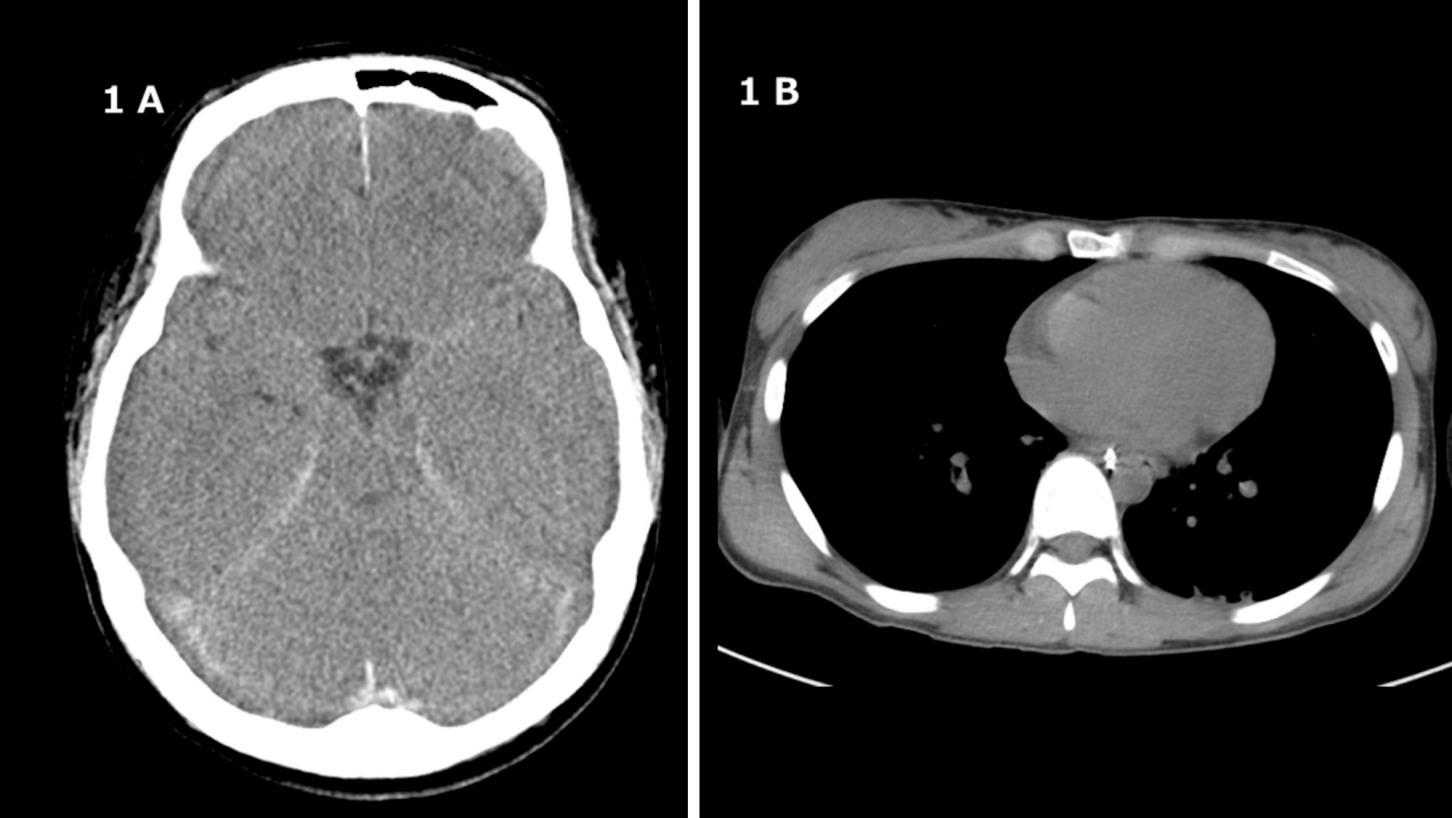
Head computed tomography shows diffuse brain edema and loss of gray-white matter contrast (A). There were no signs of air embolism in the cerebral (A) and coronary arteries (B).
